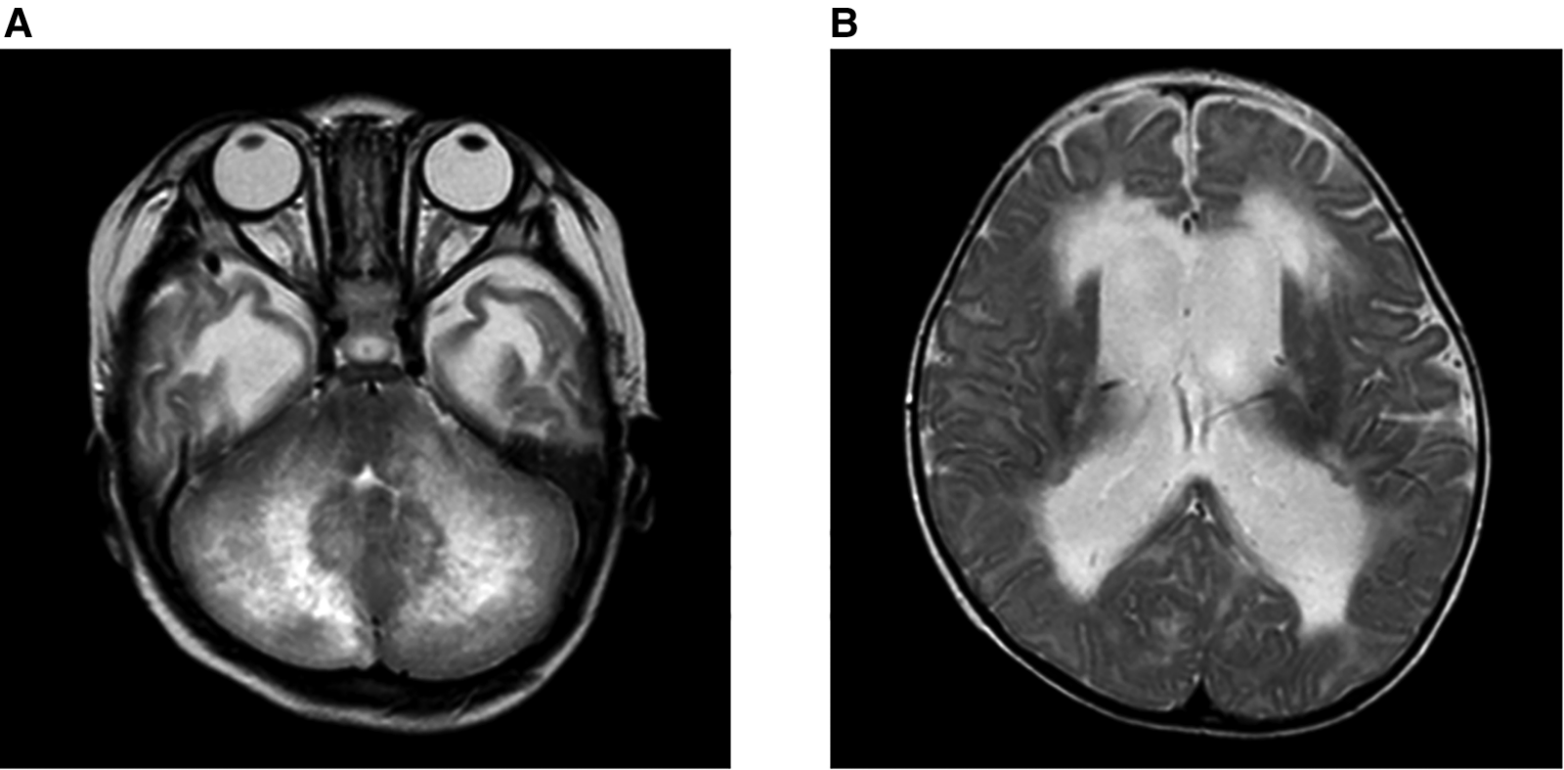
Brain MRI on admission at 7 months of age. Brain MRI on the 10 days after onset, on the T2WI. (A) Signal intensity of the bilateral cerebellar hemispheres and periventricular white matter were diffusely increased. (B) Ventriculomegaly due to the fourth ventricle obstruction. The diffuse cerebellar swelling caused the obstructive hydrocephalus.
